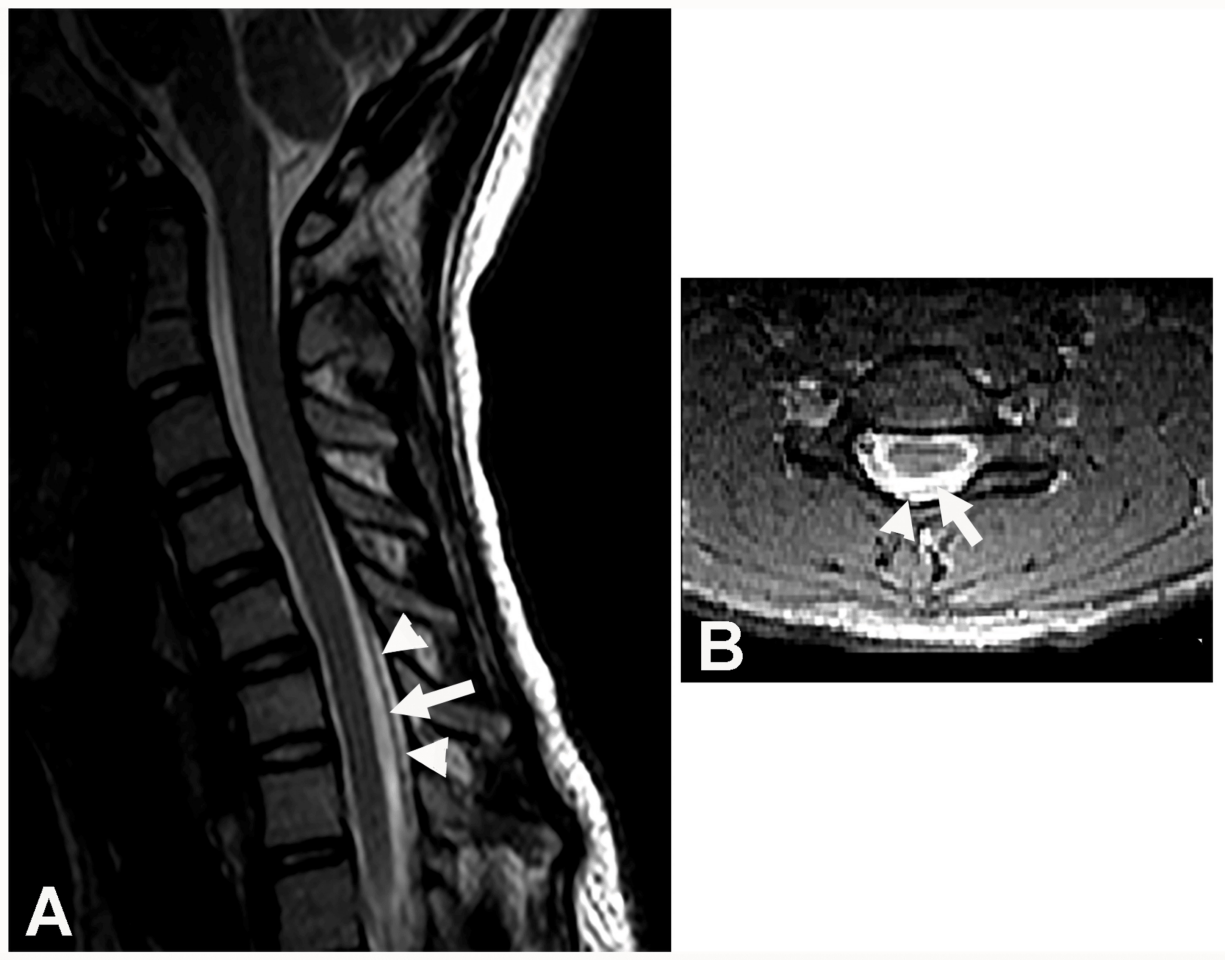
T2-weighted cervical spine MRI scan in the neutral position Case 1: T2-weighted cervical spine MRI scan in the neutral position. (A) Sagittal view demonstrating the normal position of the posterior dura (arrow), thin epidural space (arrowheads), and the normal position of the spinal cord. (B) Axial view of the cervical spine MRI scan of the lower cervical spine demonstrating the dura (arrow), normal amount of epidural fat (arrowhead), and slight atrophy of the left half of the spinal cord. MRI: magnetic resonance imaging
